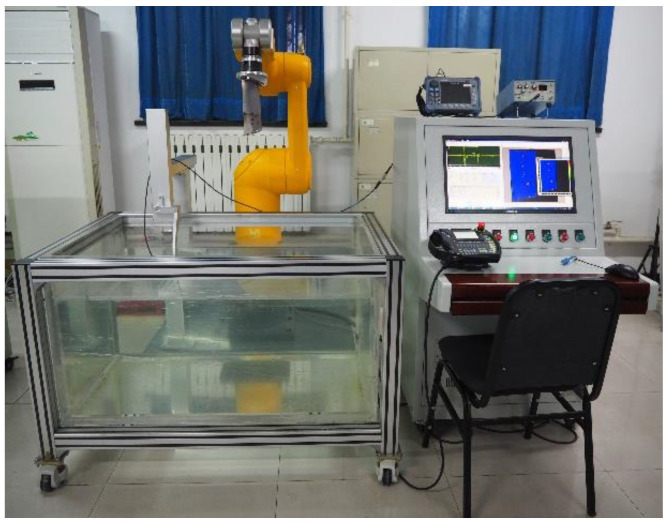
Scheme of ultrasonic nondestructive testing system for robotic.
